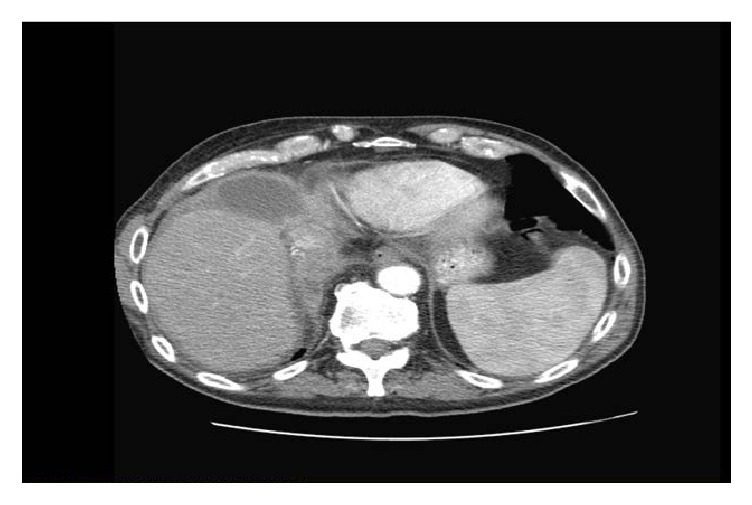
CT scan with contrast showing 5.6 cm × 2.2 cm liver mass and 3.7 cm × 2.2 cm fluid collection posterior to the anterior abdominal wall musculature.
